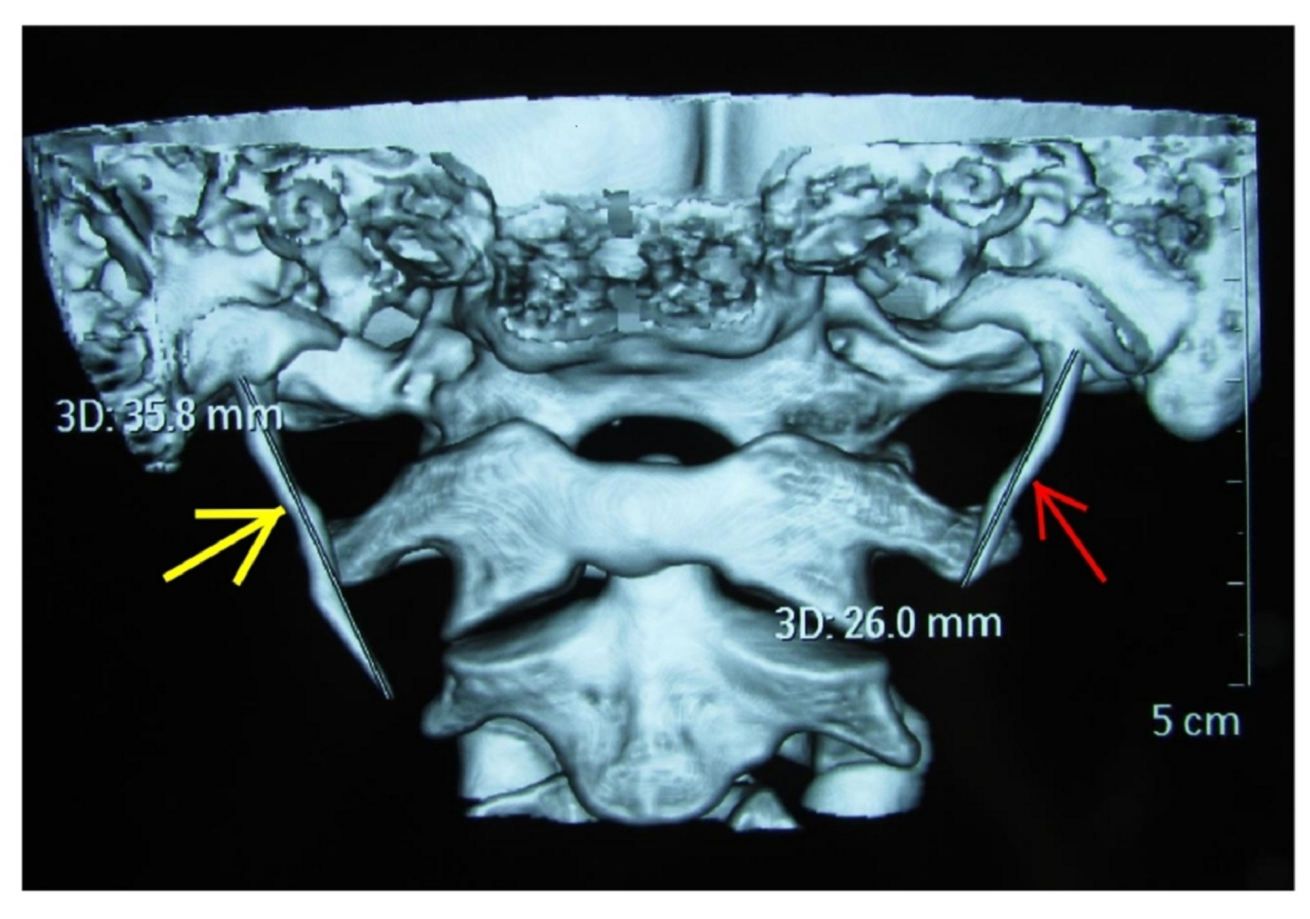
Computed tomography with three-dimensional reconstruction image revealing the length of the styloid process as 35.8 mm (yellow arrow) on the right and 26 mm (red arrow) on the left
